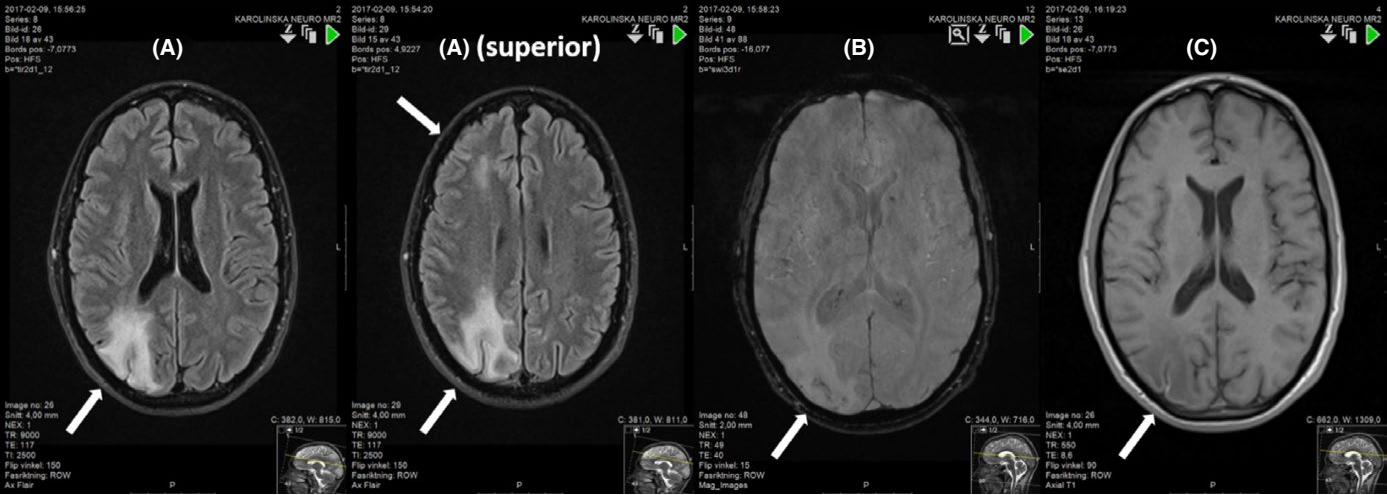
Third magnetic resonance imaging (MRI) after 3 wk (FLAIR images) when the patient was Glasgow Coma Scale (GCS 14) in the step down neurological ward. There was resolution of swelling, but widespread signal changes in the white matter in the right hemisphere interpreted as gliosis (A). The worst area of previous gyriform air also had small cortical microbleedings, (B). There were areas of cortical infarcts with laminar necrosis only affecting a thin cortical layer, and (C). The most affected areas were prefrontal, precentral gyrus, medial and posterior parietal lobe, and posterior lateral temporal and occipital lobe on the right side
